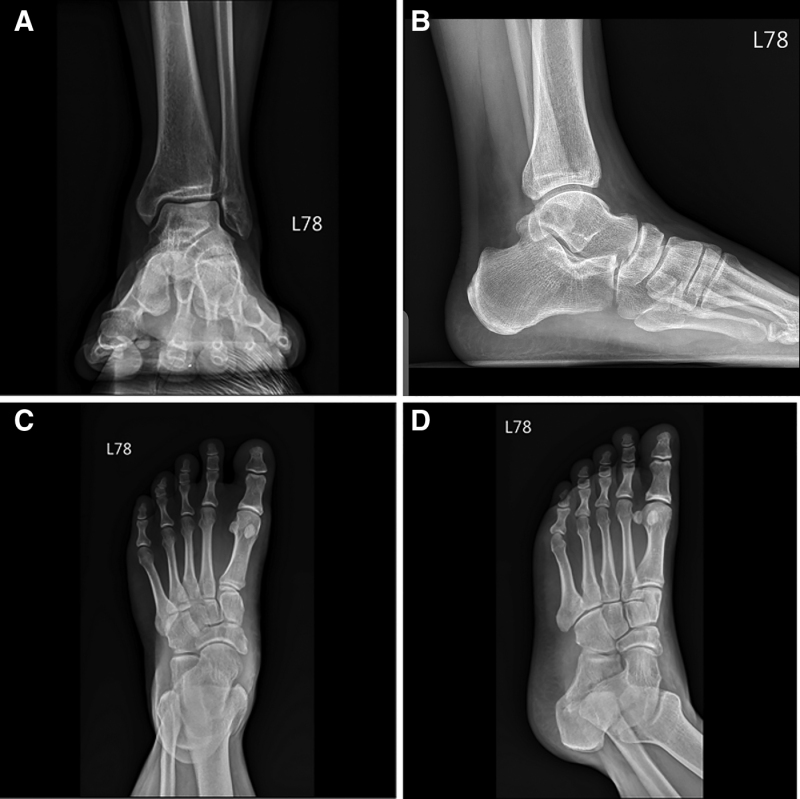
Initial plain radiographs of the left ankle and foot. Preoperative (A) anteroposterior view and (B) lateral view of the left ankle, along with (C) anteroposterior view and (D) lateral view of the left foot, demonstrating cortical irregularity of the navicular bone and a lucent lesion at its posterior facet, consistent with an osteochondral lesion.
